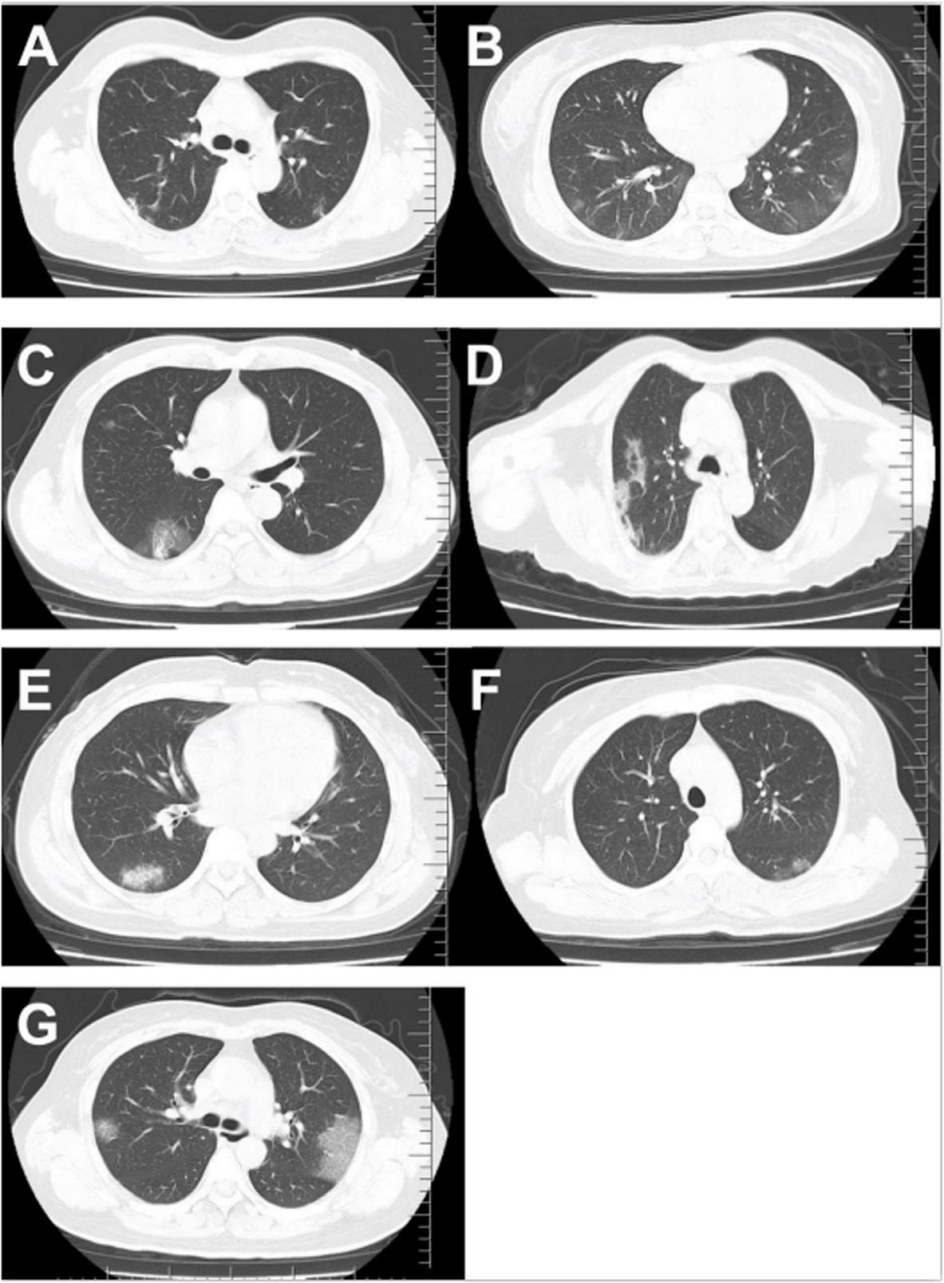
Computed tomography findings of 7 asymptomatic coronavirus disease (COVID-19) patients on admission
